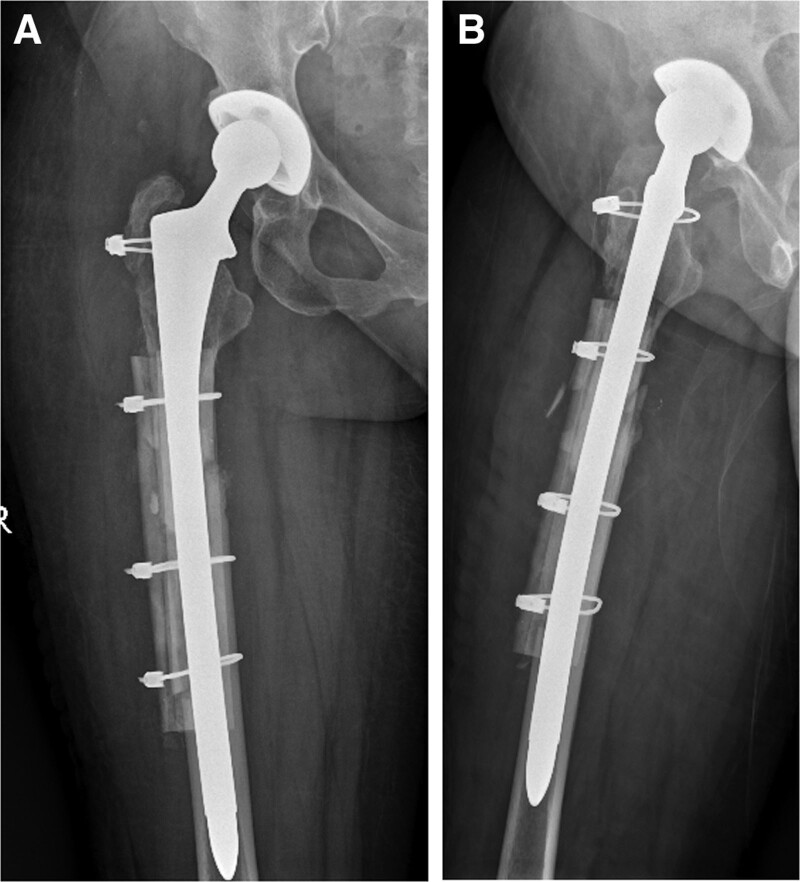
Radiographic analysis after the second revision of the right hip joint. A, B: Anterior and lateral radiographic positions after the second revision.
